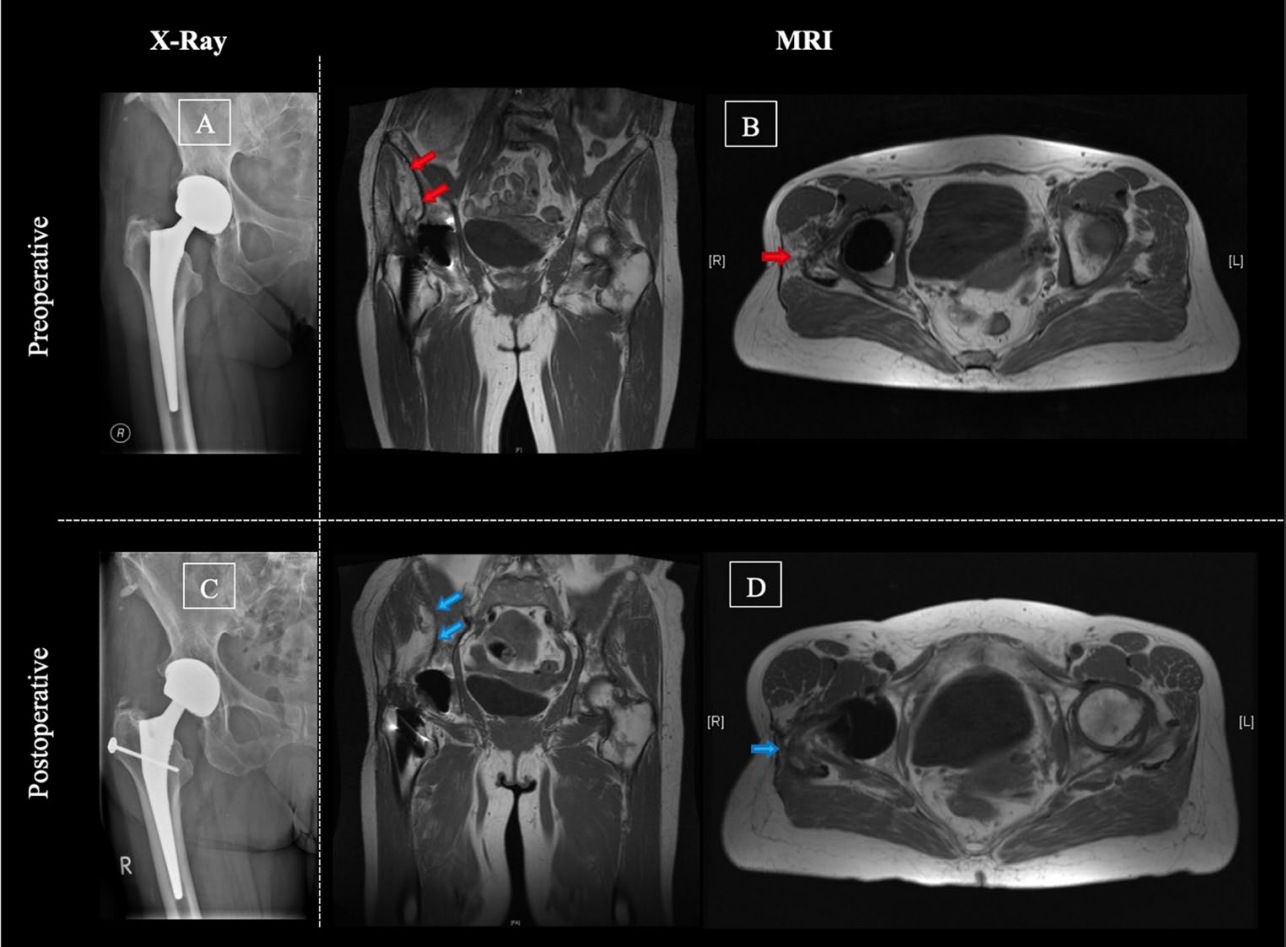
Example of pre- and postoperative radiographic evaluation. Pre- (A and B) and postoperative (C and D) X-ray and MRI images of a 69-year-old patient treated with a gluteus maximus muscle flap due to an abductor mechanism deficiency. Preoperatively, a significant degeneration of the gluteus medius muscle (red arrows) was seen without signs of component loosening or infection. The postoperative radiographic images present the screw that was used to fix the anterior flap on the proximal femur (C). The postoperative MRI shows no significant further loss of volume or degeneration of the gluteus medius muscle as well as no flap necrosis (D, blue arrows)
